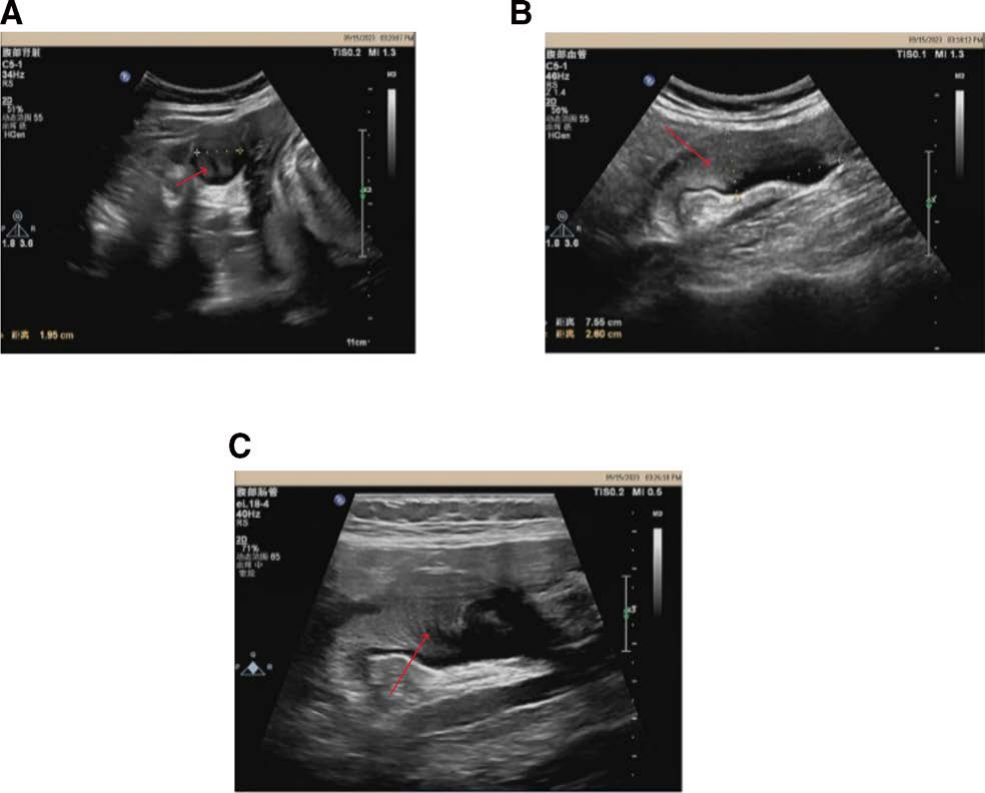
Appendix ultrasound image. (A) Transverse section of the appendix under abdominal ultrasound. (B) Longitudinal section of the appendix under abdominal ultrasound. (C) Typical “onion skin” sign on abdominal ultrasound. All red arrows indicate the typical “onion skin sign.”
